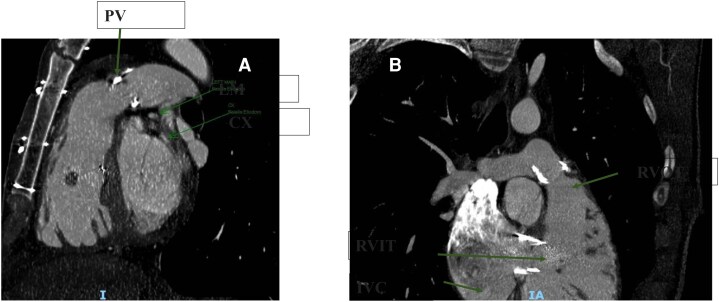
(*A*) CT (computed tomography) showing the distance between the pulmonary bioprosthesis and the coronary arteries. (*B*) CT showing the relationship between right ventricular inflow and outflow tracts. CX = circumflex artery; IVC = inferior vena cava; LM = left main; PV = pulmonary valve; RVIT = right ventricular inflow tract; RVOT = right ventricular outflow tract.
